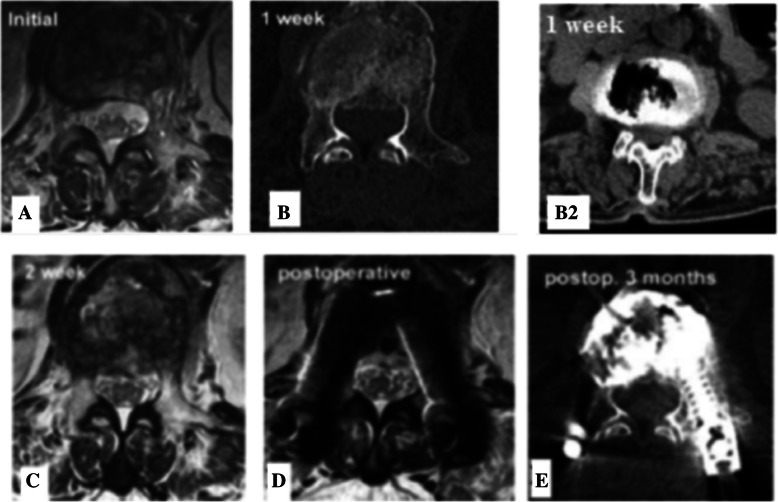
Axial MRI & CT Showing Rapid Progression and Resolution of Burst Fracture and Compression in a case of Kummell’s Disease. a Initial MRI, b1 MRI at 1 week, b2 CT at 1 week, c MRI at 2 week, d Post-operative MRI, e Postoperative CT 3 months
